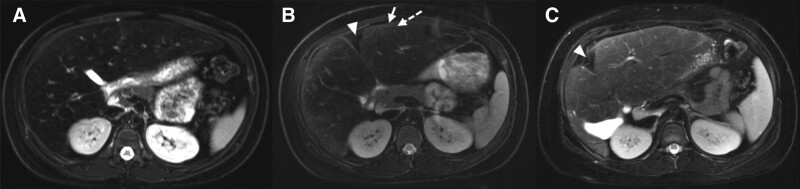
MRI abdomen T2 fat saturation axial sequences from the patient’s (A) initial MRI, (B) second MRI, and (C) most recent MRI demonstrate cirrhosis, with notable fibrosis progression from the initial to second MRI, and grossly stable imaging findings from the second MRI to the most recent MRI. The presence of subtle nodular hepatic contour (arrow), widening of the intrahepatic fissure (arrowhead), and subtle linear T2 hyperintense parenchymal bands represents areas of fibrosis (dashed arrow). Decreased fat infiltration in (C) is evidenced by the brighter signal in the liver. MRI = magnetic resonance imaging.
